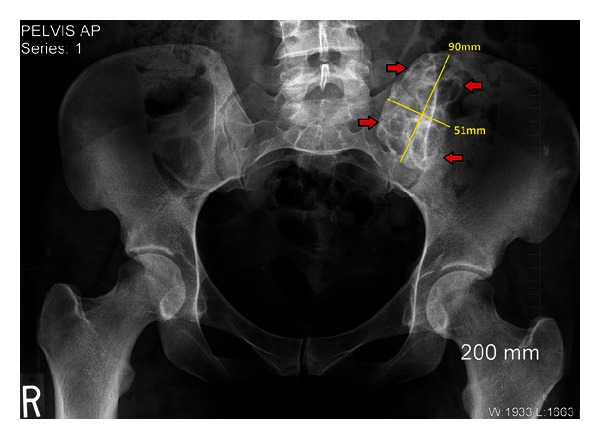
Plain radiography of the pelvis: “honeycomb” appearance—mixed lytic and sclerotic lesion (depicted within the red arrows) in the superomedial aspect of the left ilium, grossly measuring 5.1 × 9 cm.
